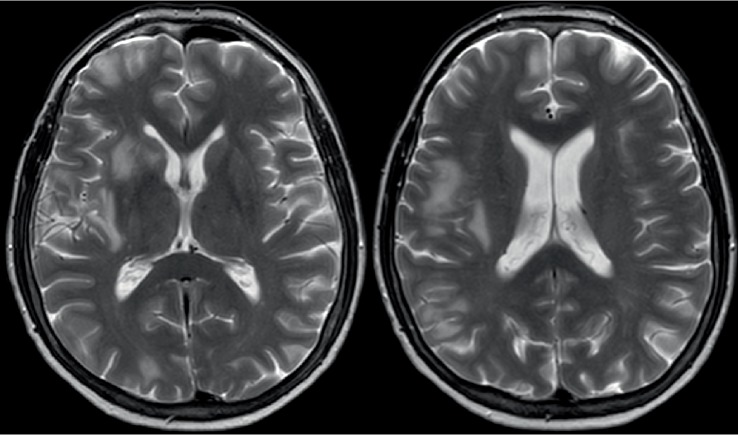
Transversal T2-weighted MR images five months after endovascular treatment show high-signal white matter changes with slight mass effect in the vascular territory of the right internal carotid artery.
